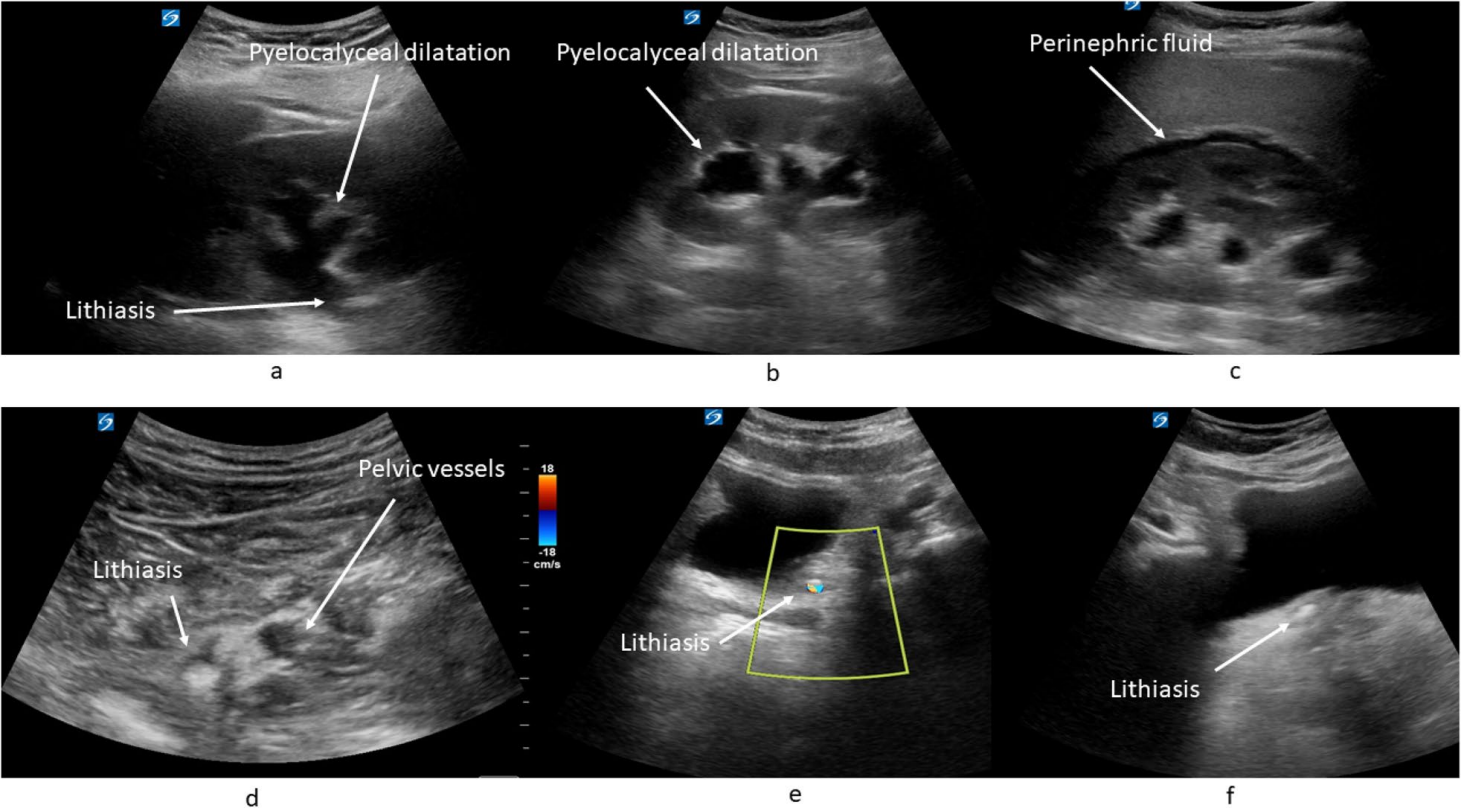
Ultrasound images: a pyelocalyceal dilatation and proximal lithiasis, b pyelocalyceal dilatation, c perinephric fluid, d pelvic lithiasis, e bladder lithiasis and twinkle artifact in color doppler; and f bladder lithiasis
